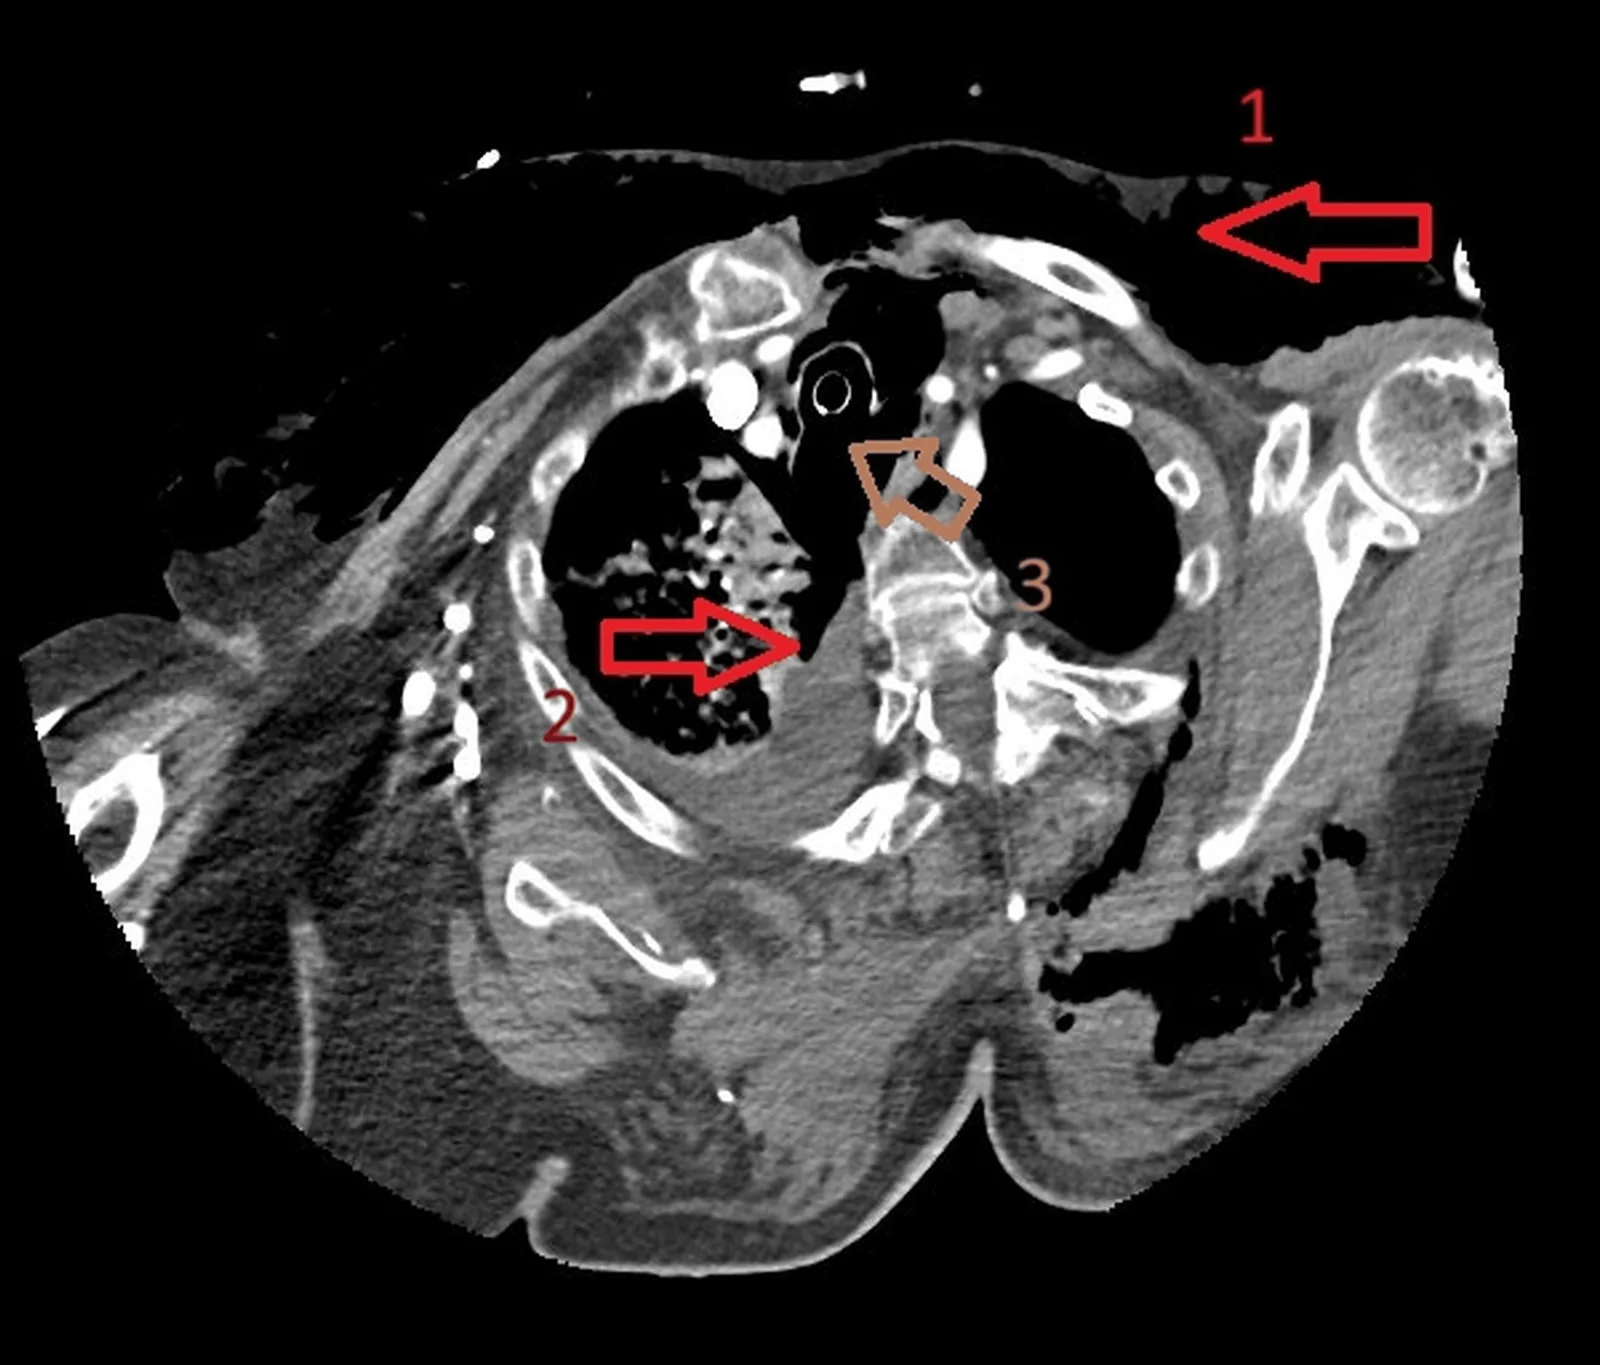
Contrast-enhanced thoracic computed tomography, axial section at the level of the thoracic inlet Subcutaneous emphysema of the anterior chest wall (arrow 1), diffuse pneumomediastinum (arrow 2), and disruption of the posterior membranous tracheal wall (arrow 3).
